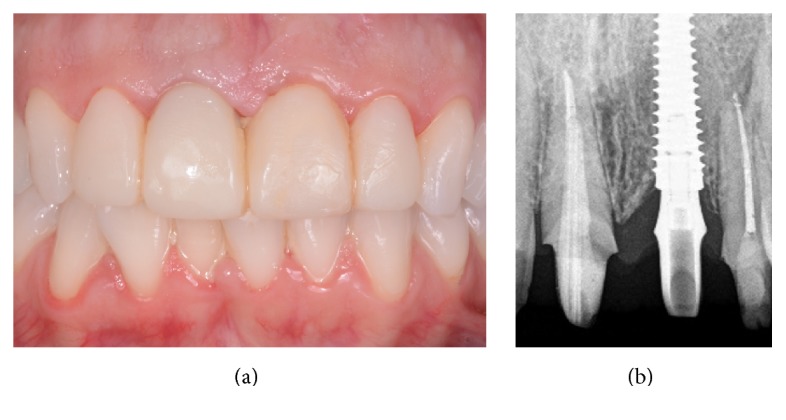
The provisional restoration after 2 months. The soft tissues outline has been modeled by the temporary and the level and curvature of the facial mucosa look better, even if oral hygiene should be improved. (a) Clinical view; (b) radiographic control.
